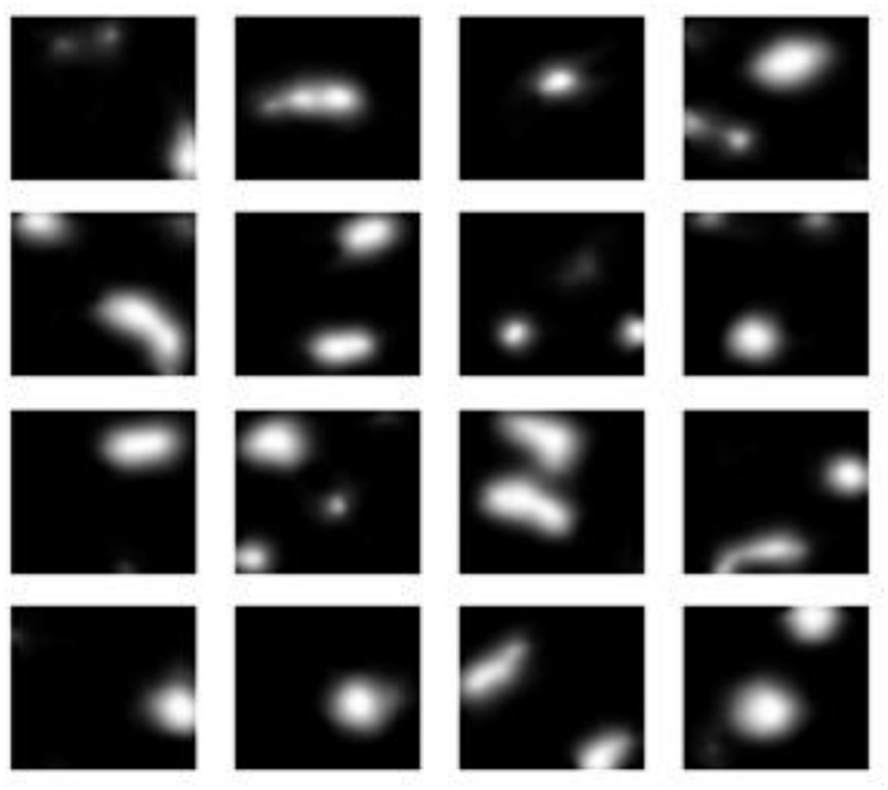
MRI generated by the StyleGAN2 model.
